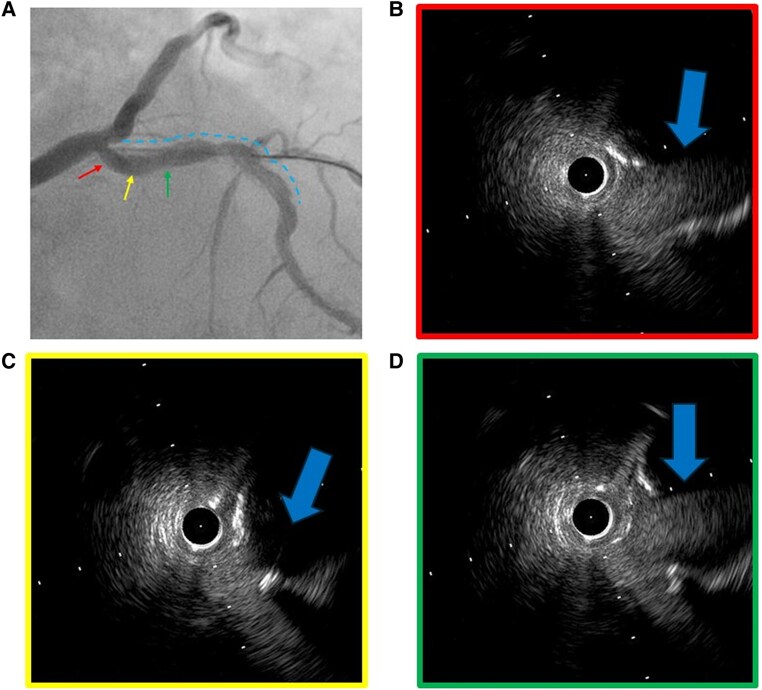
(*A*) Angiographic image following intravascular lithotripsy performed in the proximal to mid-left anterior descending artery (dashed line), showing a Type C coronary artery dissection in the proximal left anterior descending segment. Arrows indicate anatomical locations corresponding to intravascular ultrasound images. Arrows also denote the true lumen. (*B*) Intravascular ultrasound image showing medial disruption and migration of both the wire and intravascular ultrasound catheter into the subintimal space. (*C*) Intravascular ultrasound image demonstrating the wire and catheter located behind the calcified plaque, within the subintimal space. (*D*) Intravascular ultrasound image confirming the wire’s position in the subintimal space adjacent to the true lumen.
